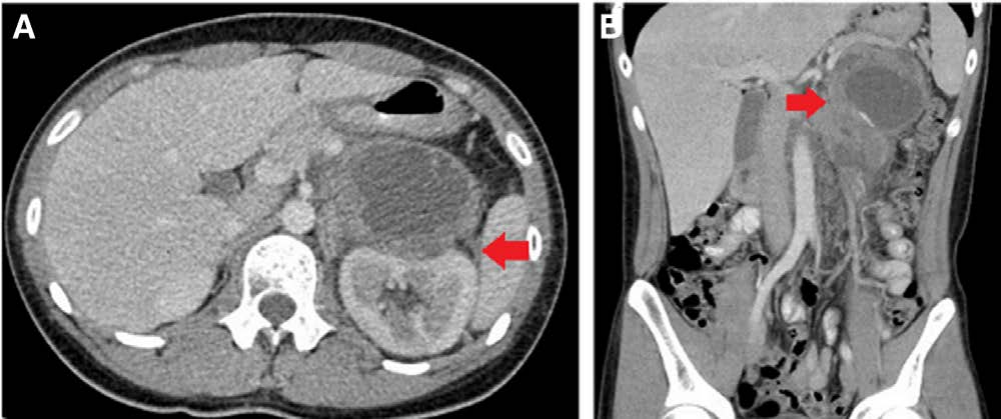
(A, B) Abdominal computed tomography. A heterogeneous mass with calcification and cystic and solid components was found in the pancreatic tail compressing the left kidney (arrow).
